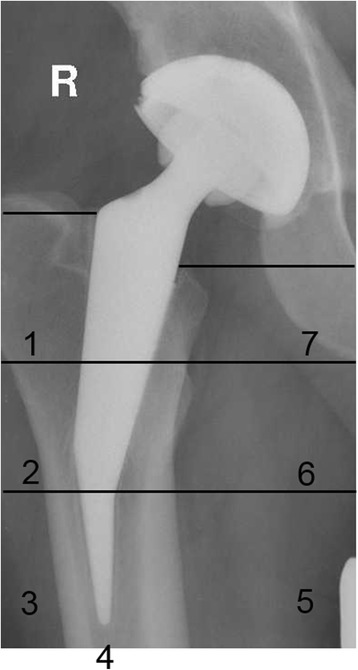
Radiographic appearance of linear radiolucencies in the zones 1, 3 and 5 of Gruen
